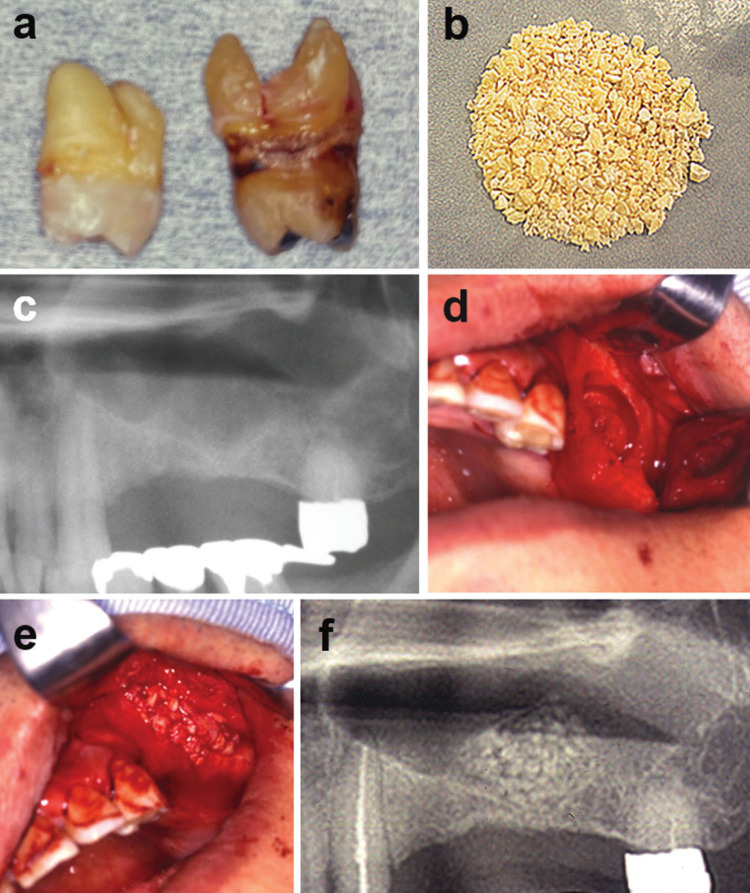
a,b: Preparations of partially demineralized dentin matrix (DDM). a. extracted molars (tooth #17, #18). b. freeze-dried DDM (total weight:2.06g). c-f: DDM autograft for sinus lifting in 48-year-old man. c. X-ray photo before sinus lifting. Note: 3 missing teeth (tooth #24,#25,#26) and atrophic maxilla. d. oval-shaped bone window. e. autogenous DDM graft. f. X-ray photos at 1 day after DDM graft. Ununiform of radio-opacity by partially demineralized dentin granules.
